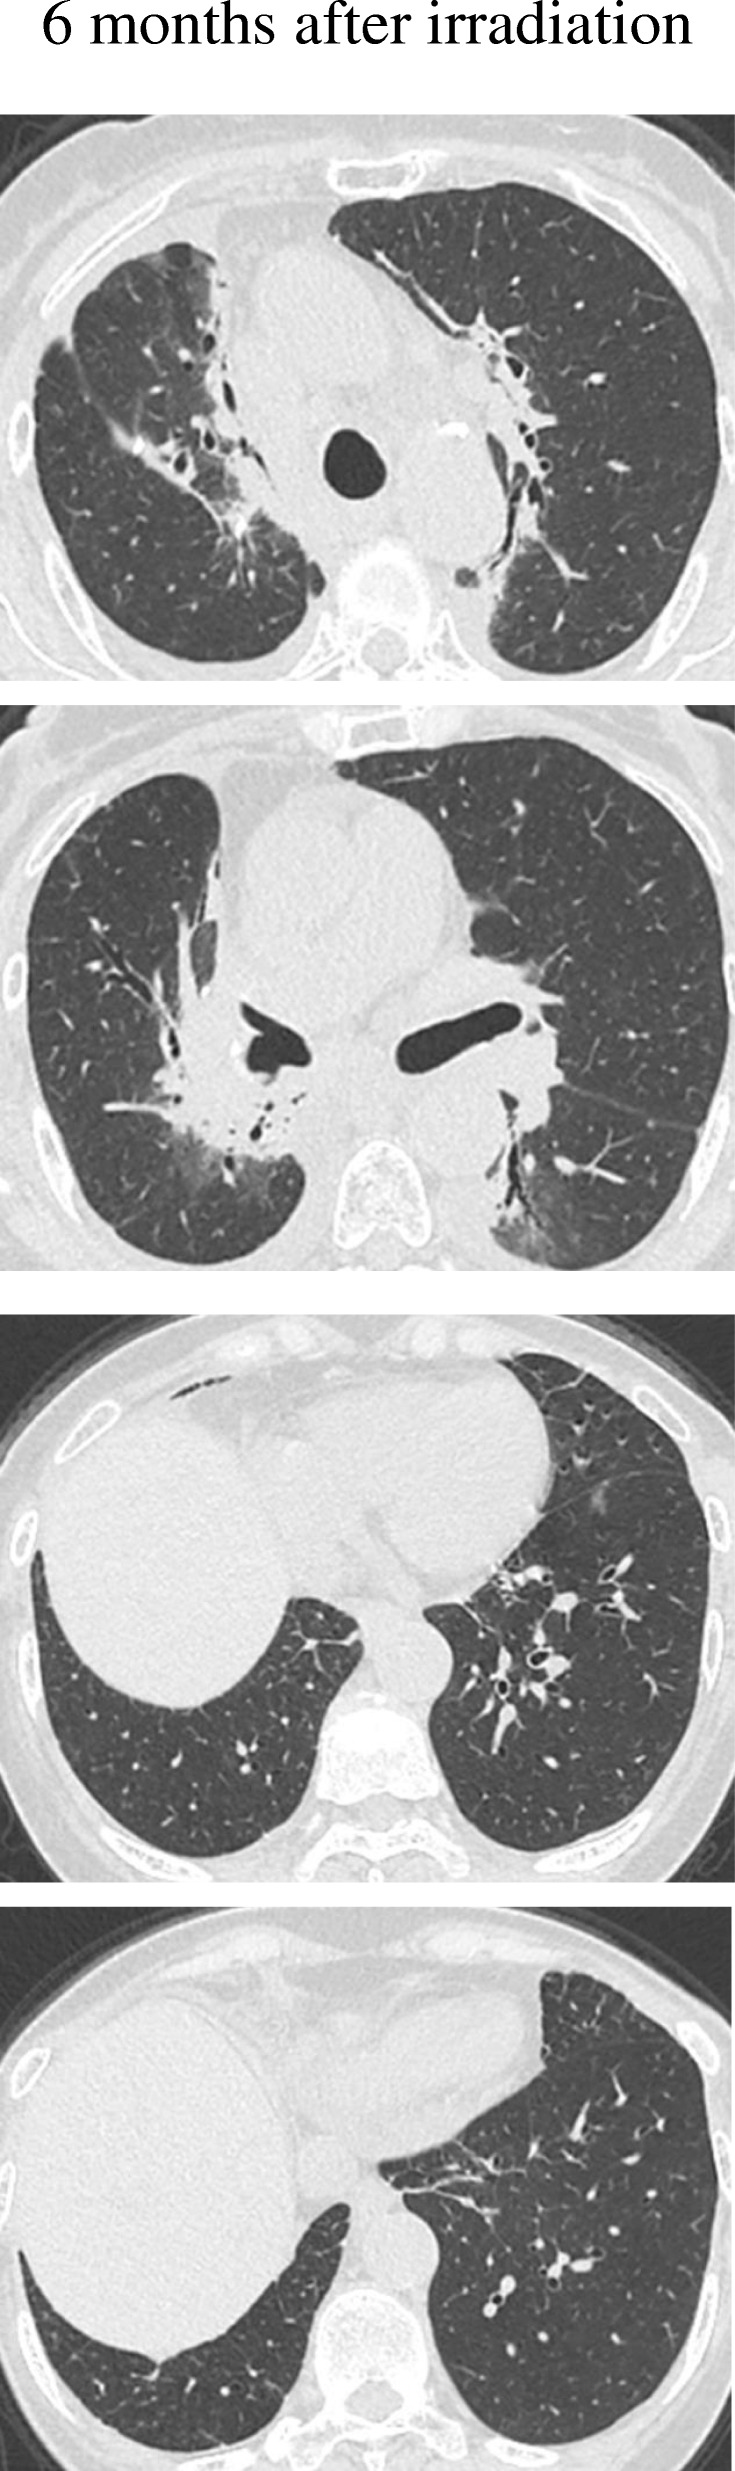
A follow-up computed tomography scan of the chest, performed 6 months after the irradiation therapy, showed radiation pneumonitis but no reappearance of pulmonary metastases in either lung
